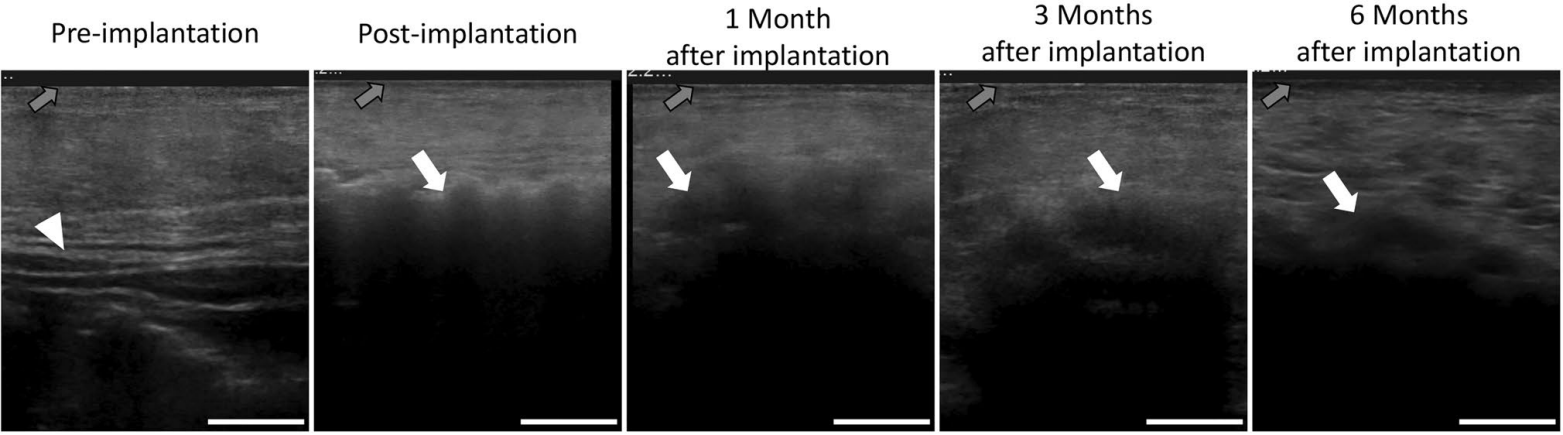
Ultrasound evaluation of the implant aggregate. The outer surface of the implant aggregate was defined immediately after implantation, but became less distinguished over time. The acoustic shadow of the outer surface diminished over time. The hyperechoic area was confirmed in the internal space of the implant aggregate 1 month after implantation. The white arrowhead indicates the deep fascia. The white arrows indicate the outer surface of the implant aggregate. The gray arrows indicate the epidermis. Scale bar: 1 cm
